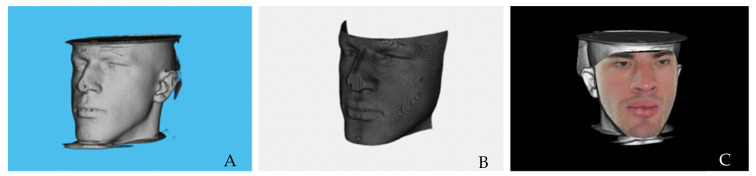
Combination of stereophotogrammetry images with CBCT images to create 3D virtual models of the patient: untextured soft tissue surface of the CBCT scan (A,B). Fusion of a CBCT soft tissue image with 3D stereophotographic images (C).
